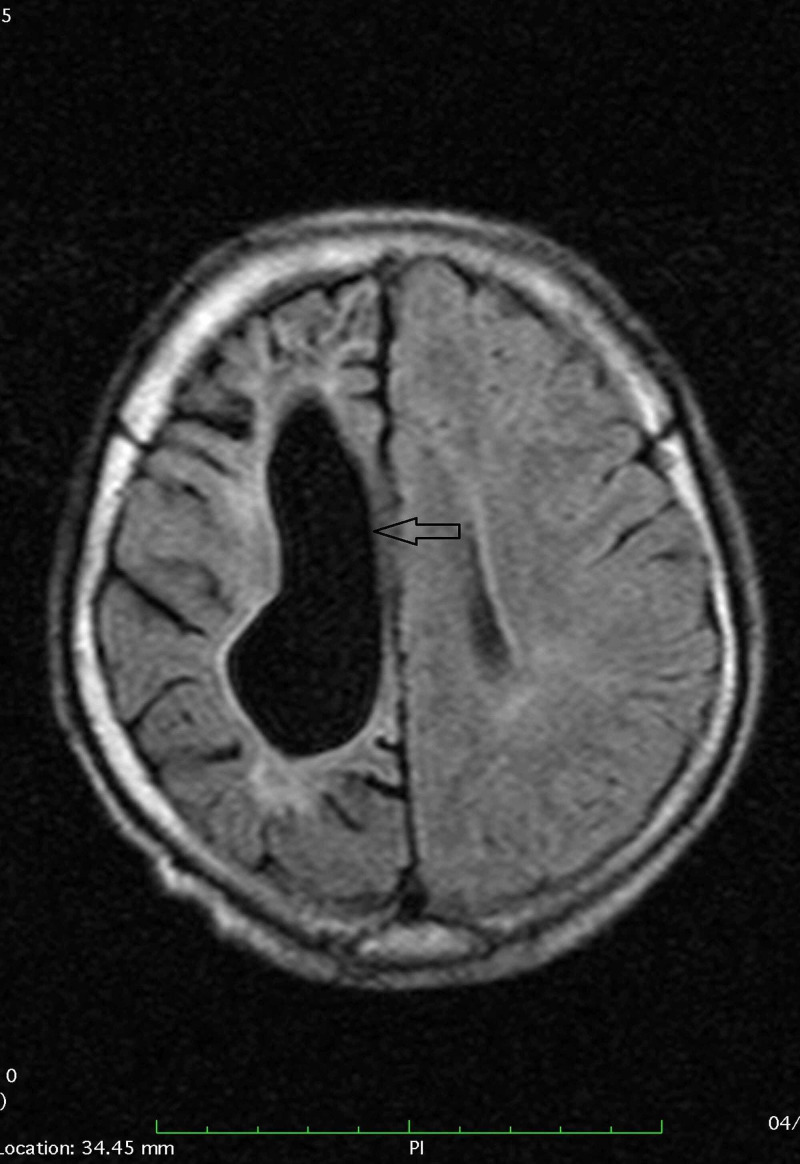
CT scan image showing the atrophy of the right cerebral hemisphere with the prominence of extra-axial cerebrospinal fluid (CSF) spaces (black arrow)
